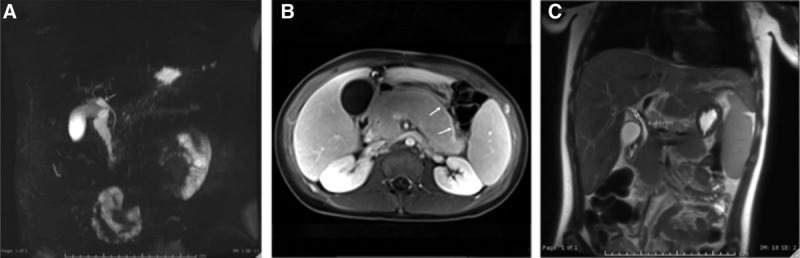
Magnetic resonance cholangiopancreatography (MRCP) of patients 1, 2, and 3. A, Patient no. 1 is a coronal 3D MRCP image. B, Patient no. 2 is an axial T1 post-contrast delayed (120 seconds) image, and (C) patient no. 3 is a coronal single-shot fast spin-echo image. Provided MR images display effects of chronic autoimmune pancreatitis, including dilatation of the CBD, delayed pancreatic capsular enhancement, and patchy parenchymal enhancement. Additional characteristic features of AIP with full descriptions found in Table 1.
